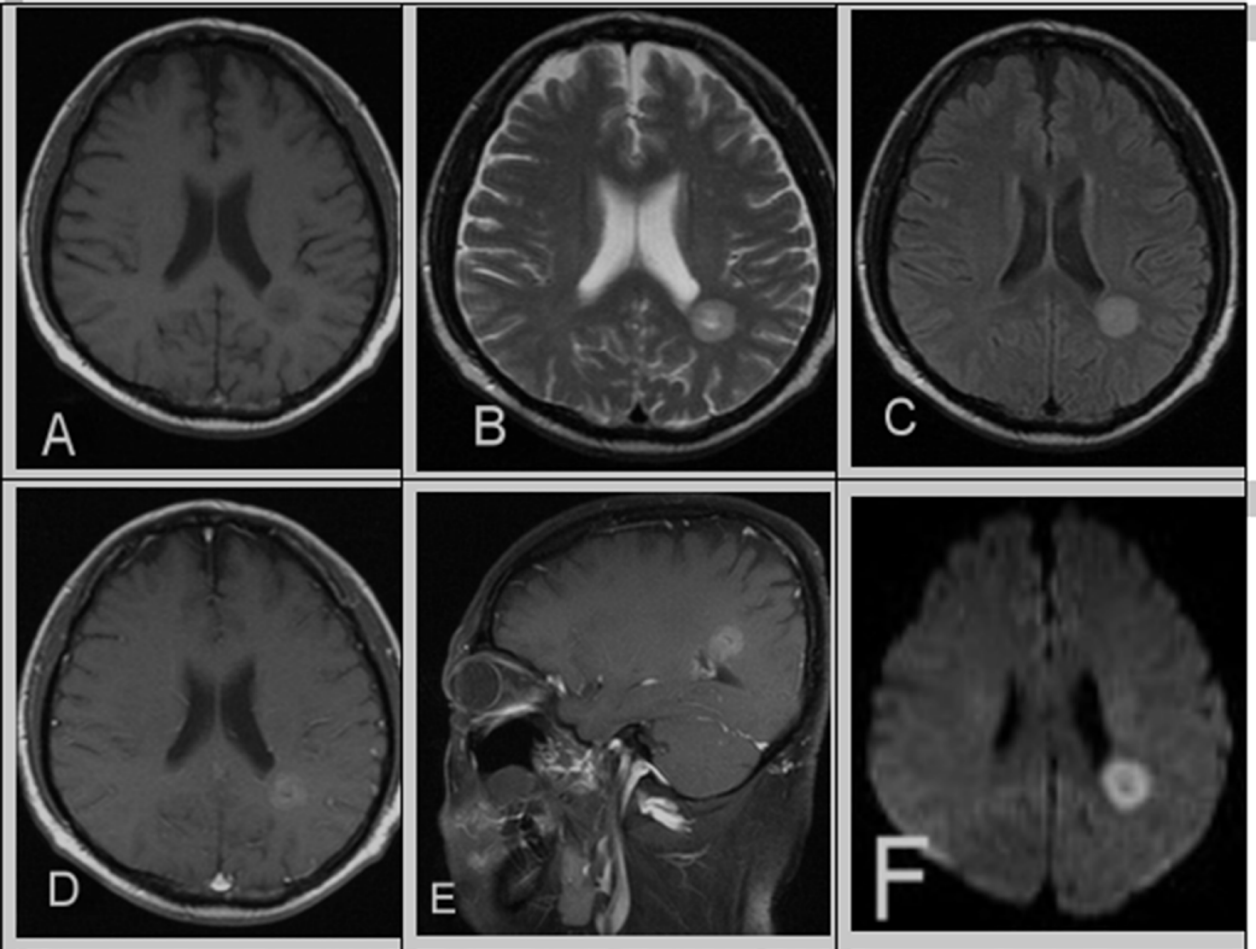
A 39-year-old male with a 10-year history of CML in relapse presents with headaches (A) Unenhanced T1WI revealed a well-defined, heterogeneous brain parenchyma mass with mild hypointensity. (B) T2WI and (C) FLAIR showed hyperintensity, post-contrast. (D, E) T1WI showed mild hyperintensity and cystic changes. (F) DWI showed evidence of restricted diffusion in the corresponding area. There was no surrounding vasogenic edema or mass effect.
